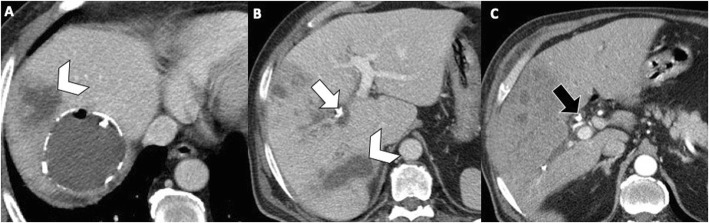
A 57-year-old man with communicating rupture and superinfection of liver hydatid cyst. Axial contrast-enhanced CT images at different levels (a, b) show multiple intrahepatic abscesses (arrowheads), adjacent to a hydatid cystic lesion with peripheral wall calcifications and intracystic air content. Note a calcified fragment of wall cyst within the right intrahepatic bile duct (white arrow), which migrates into the common bile duct (black arrow) at 10-days CT follow-up (c)
